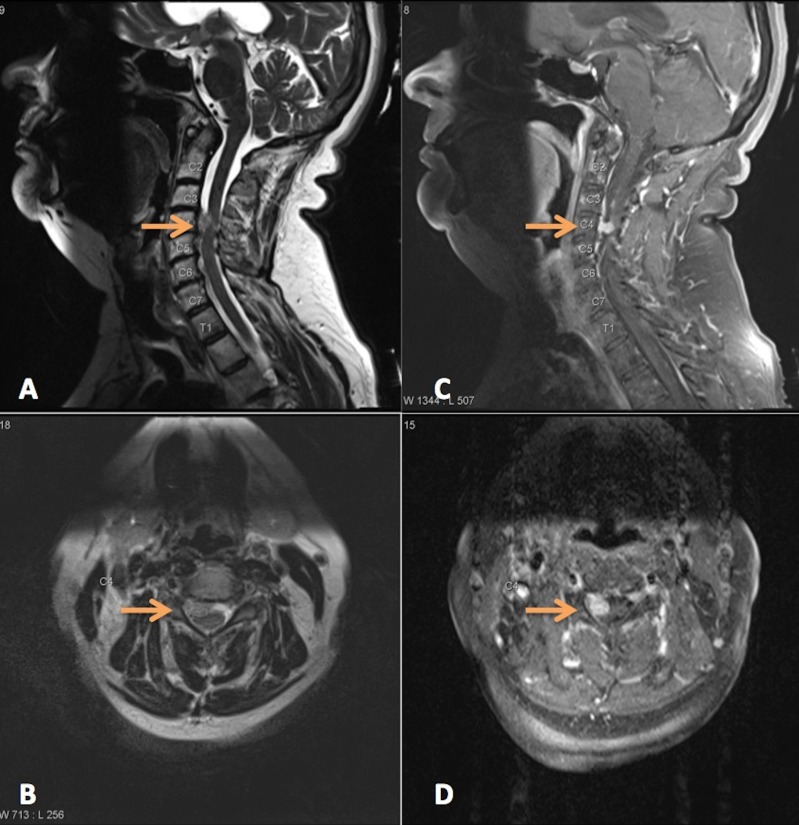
Preoperative MR Imaging A) Preoperative T2 sagittal MRI shows ventral C4-5 intradural mass w/ cord compression B) Preoperative T2 axial MRI shows right sided displacement of C5 nerve root C) Preoperative T1 sagittal MRI with contrast shows avid enhancement D) Preoperative T1 axial MRI with contrast shows avid enhancement
